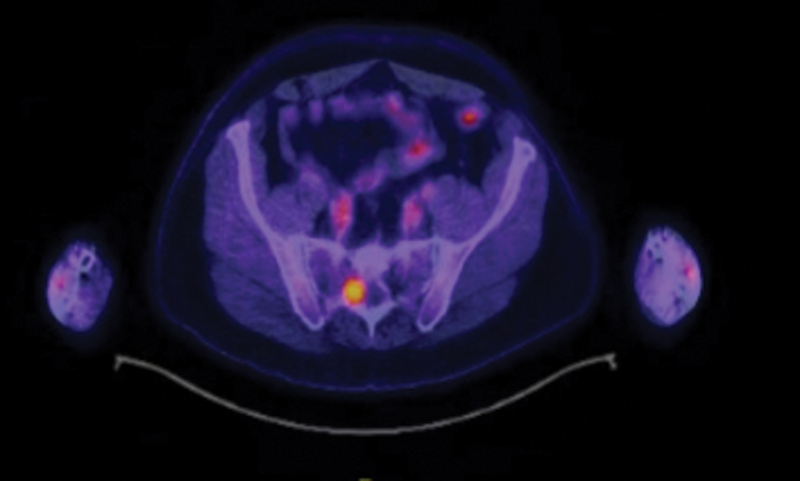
PET/CT image showed increased FDG uptake in the right S2 nerve root.
